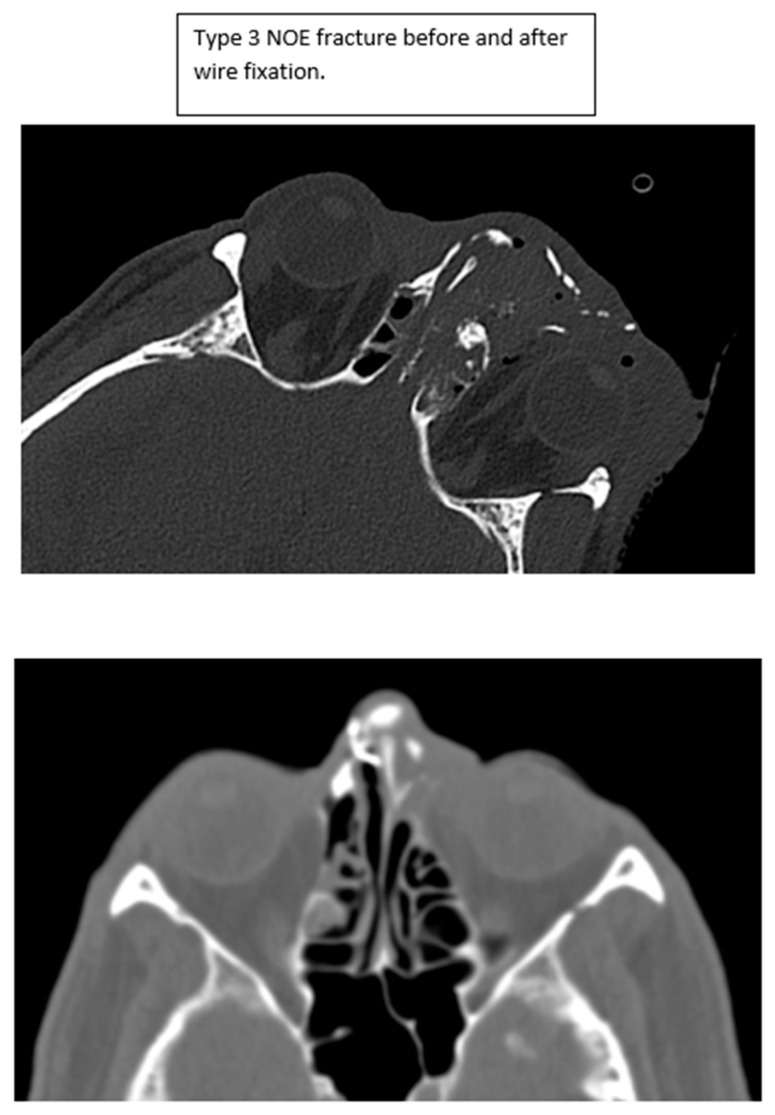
Type 3 NOE fracture CT imaging before and after intercanthal wiring.
